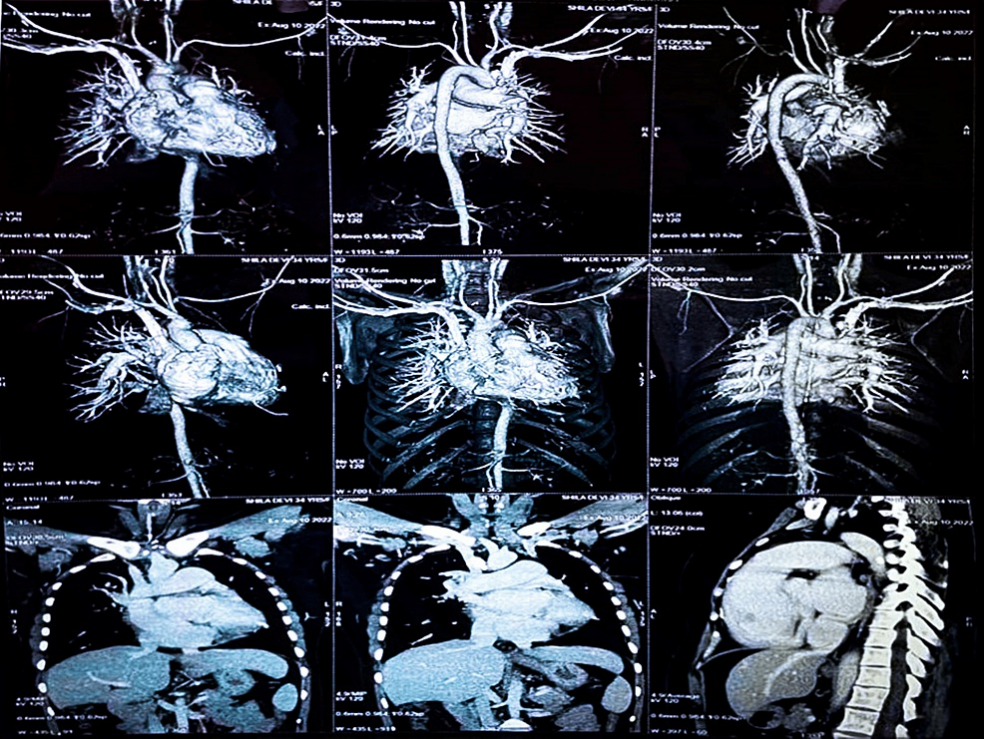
Follow-up CT angiography findings suggest mild cardiomegaly with relative enlargement of the right-side chambers and the left hepatic vein draining into the coronary sinus, along with persistent left superior vena cava draining into the coronary sinus
